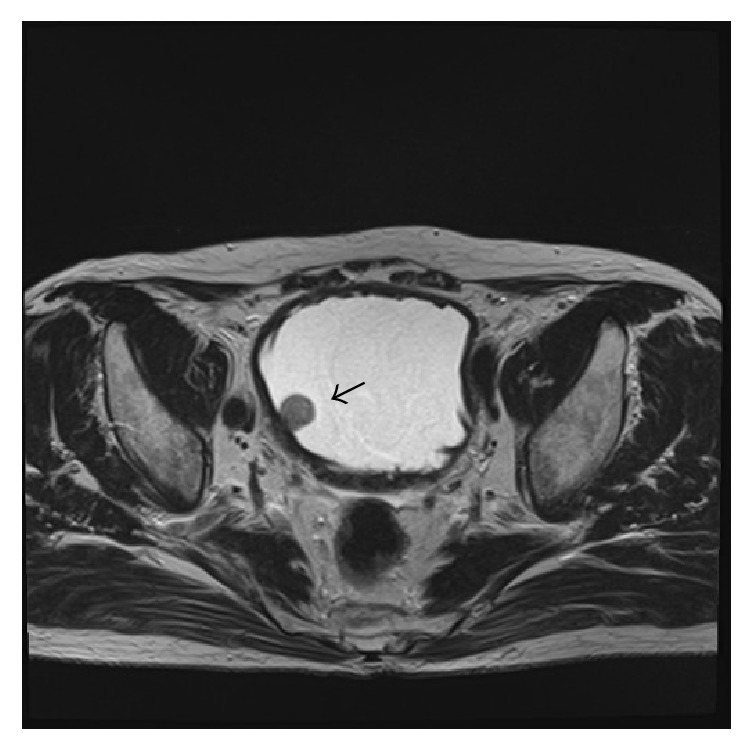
Pelvic MRI. Pelvic MRI T2 showed a 2.5 cm relatively homogenous mass (allow) in the right lateral bladder wall without extending base of the bladder wall.
